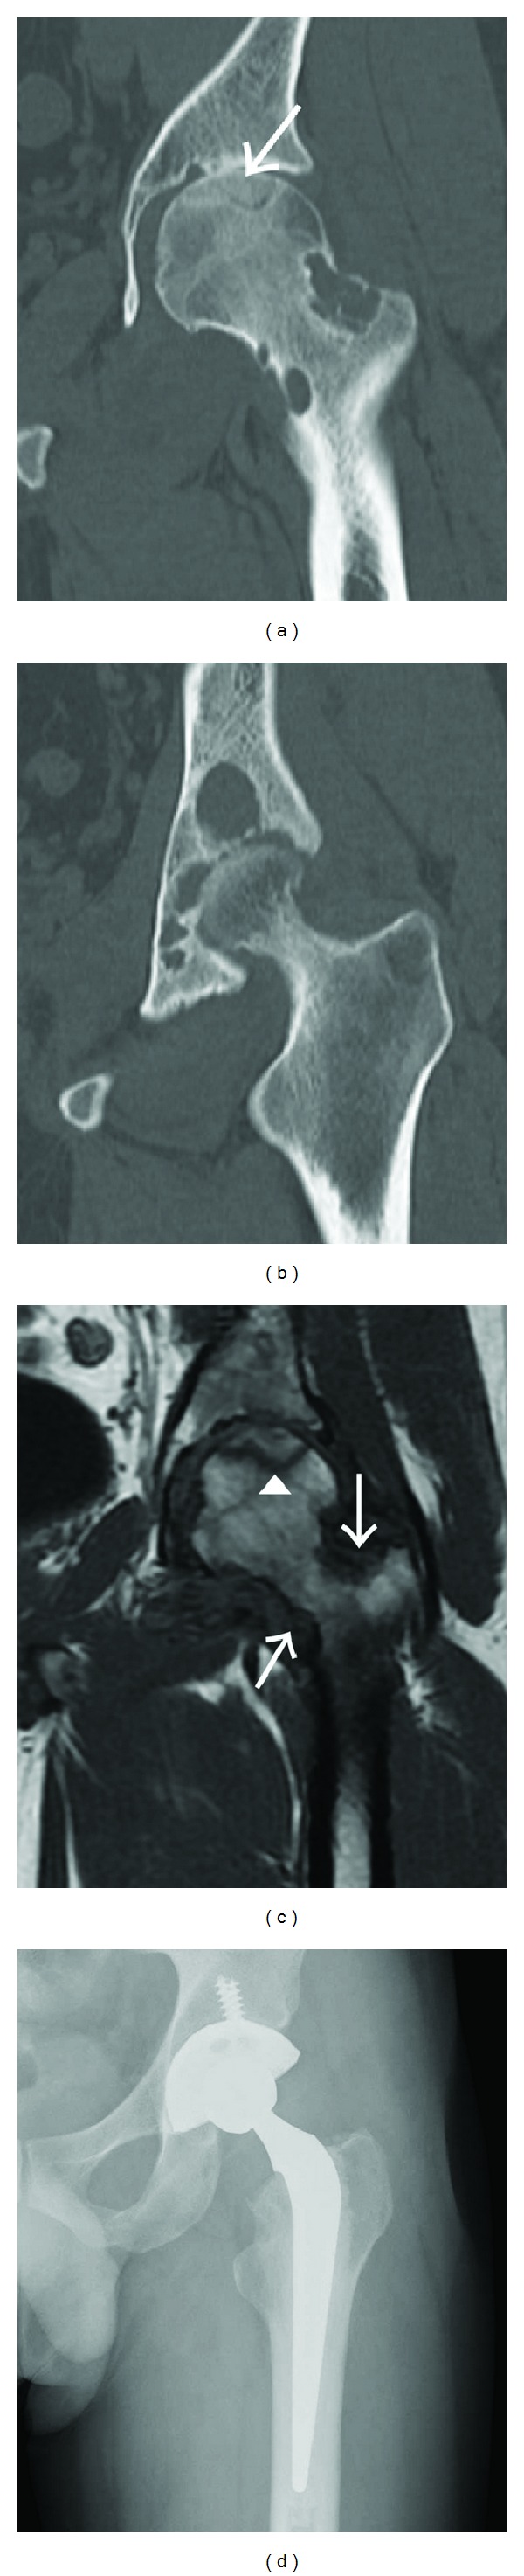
(a, b) Computed tomography image showing the femoral neck and acetabular bone erosions, and area of bone necrosis (arrow). (c) Magnetic resonance image showing a typical low-intensity band of osteonecrosis of the femoral head (arrowhead) and mass lesions (arrows) around the femoral neck on T1-weighted imaging. (d) The patient underwent total hip arthroplasty with complete synovectomy and tumor resection using an argon laser.
